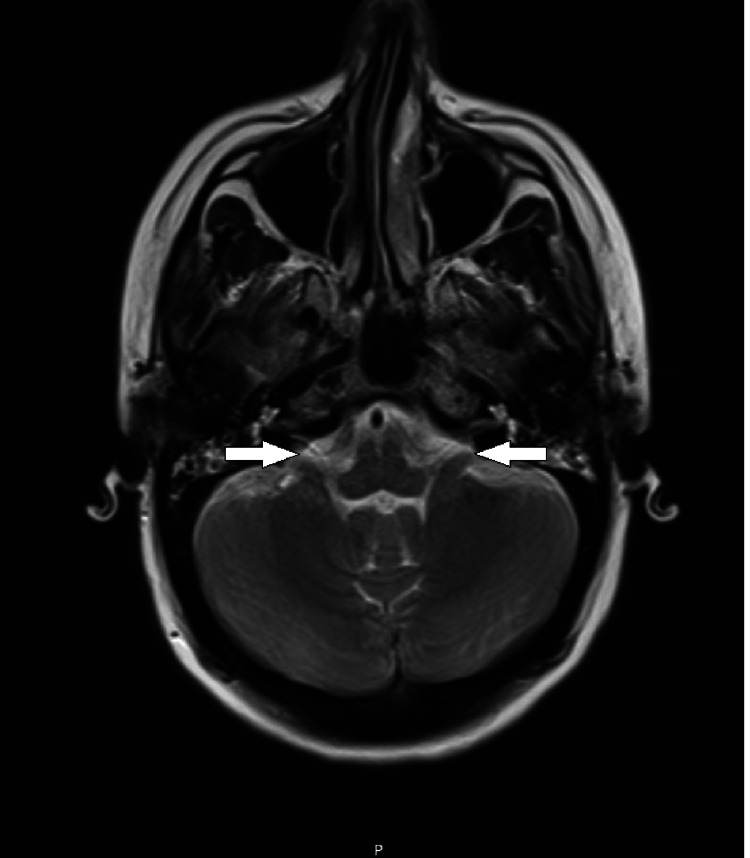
T2 weighted MRI of brain completed on post-op day #12. Imaging with enhancement of bilateral vestibular cochlear nerves consistent with encephalomeningitis. Arrows detail enhancements bilaterally.
